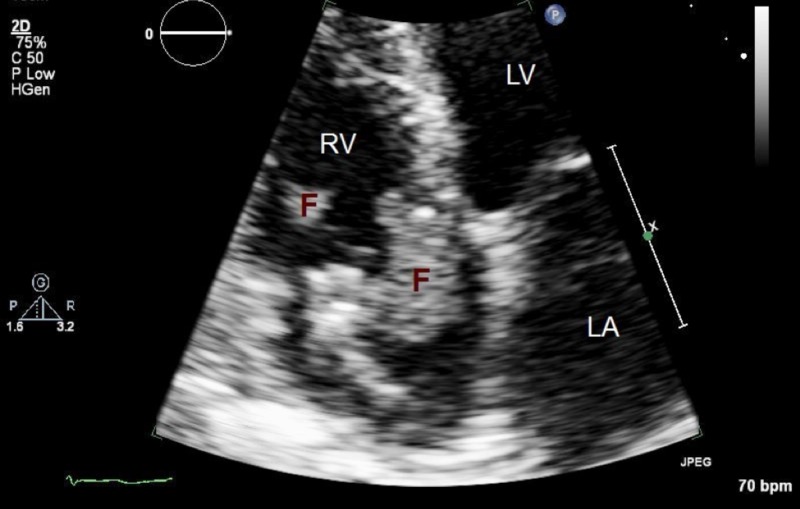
Trans-thoracic echocardiogram TTE showing a large 2 x 2-cm sessile mass (F) attached to the tricuspid valve which prolapsed into the right atrium during systole. TTE: trans-thoracic echocardiogram
